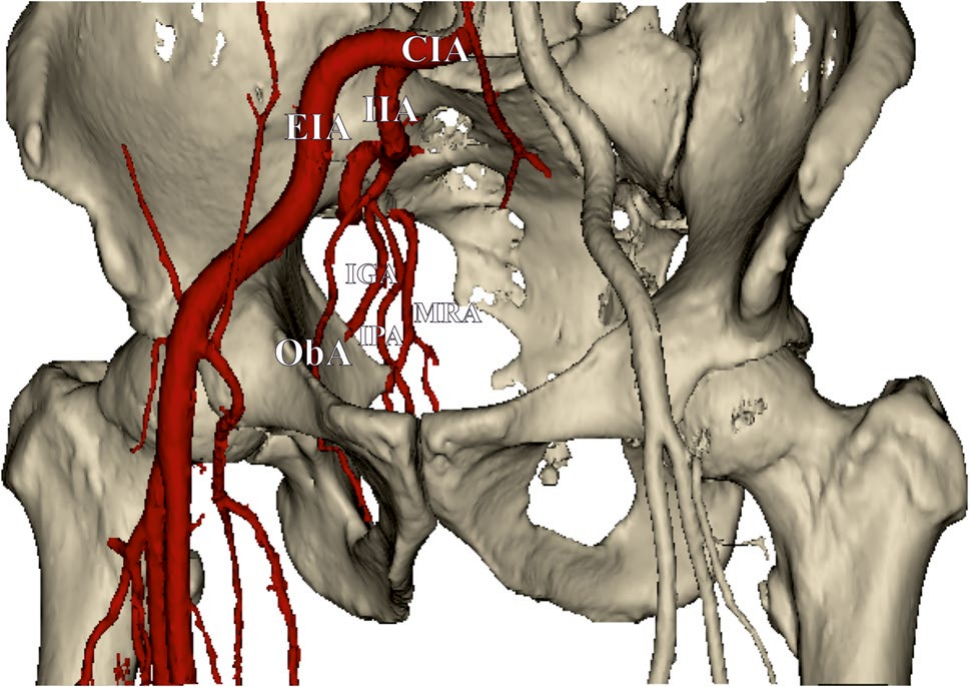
Three-dimensional, computed tomography angiography of the studied area. Some of the arteries were removed in order to provide better visibility. *ObA* obturator artery, *CIA* common iliac artery, *EIA* external iliac artery, *IIA* internal iliac artery, *IGA* inferior gluteal artery, *MRA* middle rectal artery, *IPA* internal pudendal artery
